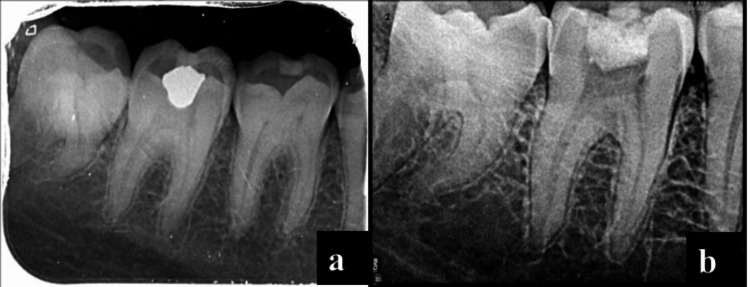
Preoperative radiograph (a); access opening radiograph (b)
